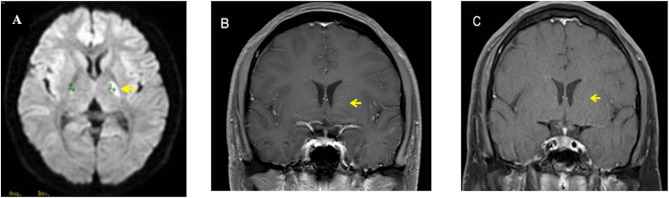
Brain MRI findings at different disease stages. (A) Axial diffusion-weighted image obtained during the first episode (June 2020) showing a hyperintense lesion in the left basal ganglia (arrow), initially misinterpreted as acute ischemic stroke. (B) Coronal contrast-enhanced T1-weighted image obtained during the second episode (August 2020) revealing characteristic radial linear perivascular enhancement perpendicular to the lateral ventricles (arrow), a hallmark of autoimmune GFAP astrocytopathy. (C) Coronal contrast-enhanced T1-weighted image obtained 3 months after immunosuppressive therapy (November 2020) demonstrating complete resolution of the previous periventricular enhancement.
